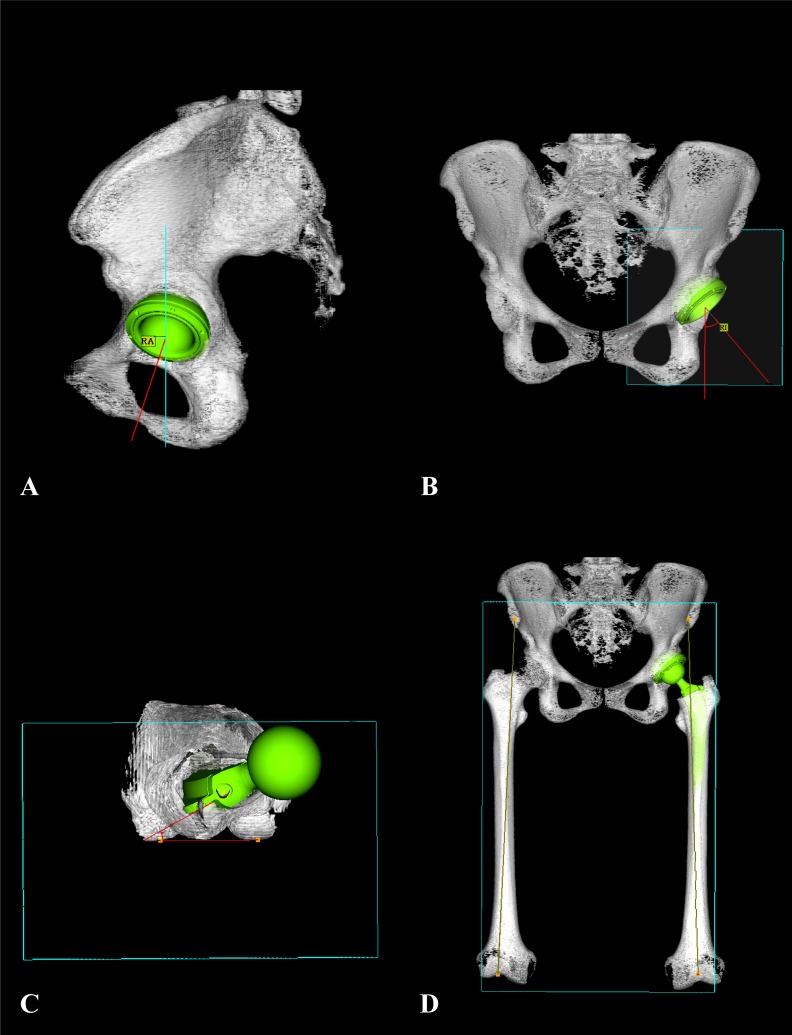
Postoperative cup and stem alignment as well as change in leg length were assessed using CT with 3D-Template system. A: Cup anteversion, B: Cup inclination, C: Stem anteversion, D: Leg length.
